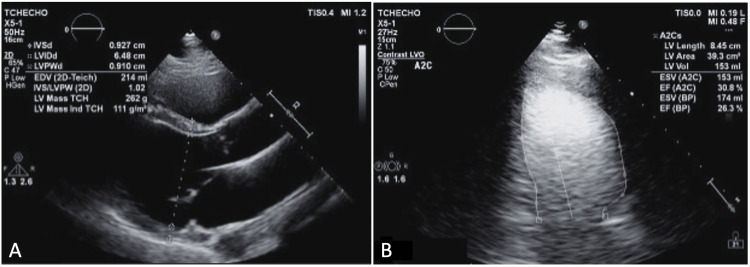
Parasternal long-axis (A) and two-chamber view (B) TTE demonstrates a dilated LV cavity with end-diastolic diameter measuring 6.5 cm; however, relative wall thickness and mass index are normal. LVEF of 26% using the Simpson biplane method. TTE: transthoracic echocardiography; LV: left ventricular; LVEF: left ventricular ejection fraction
